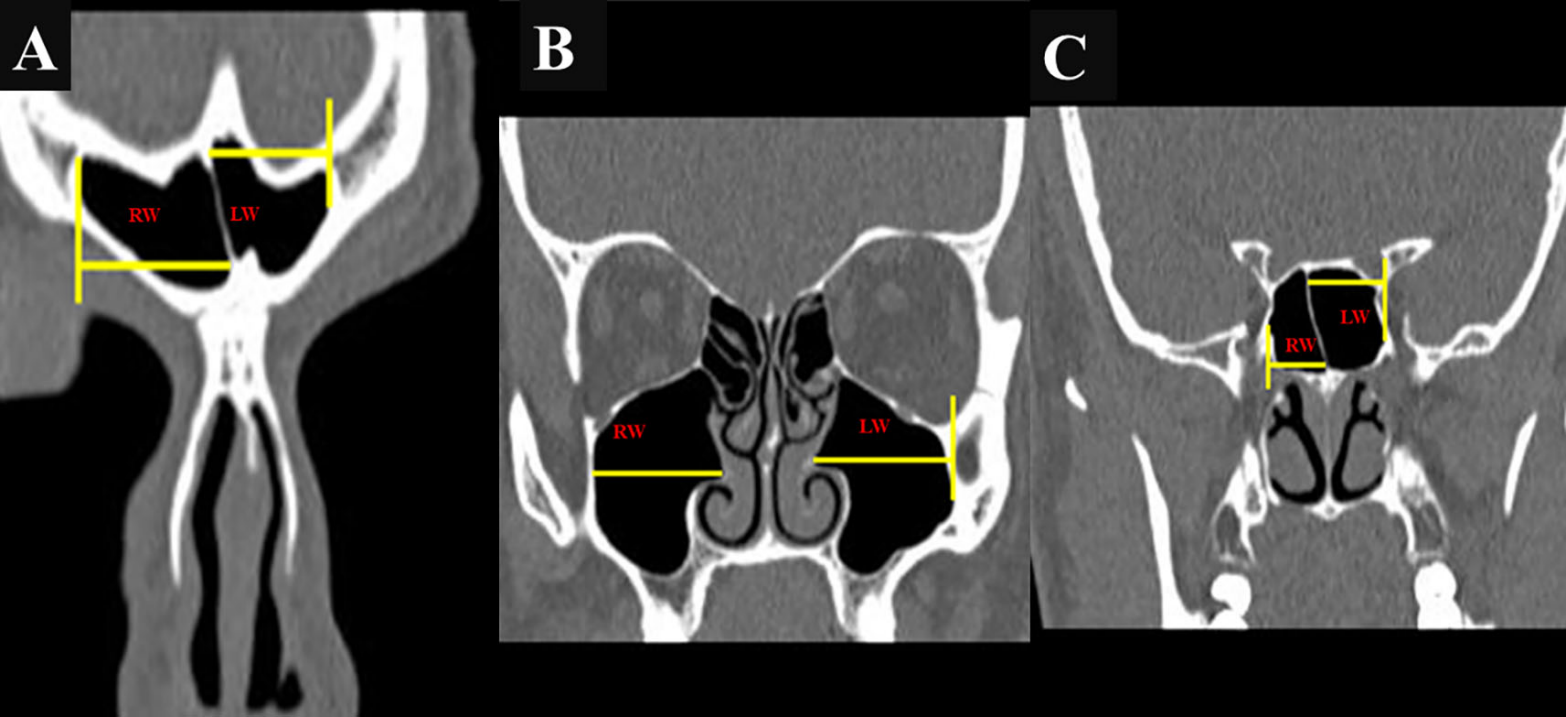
(A, B, C): Coronal CT image showing width measurements of FS, MS, and SS, respectively. (RW: right width; LW: left width).
